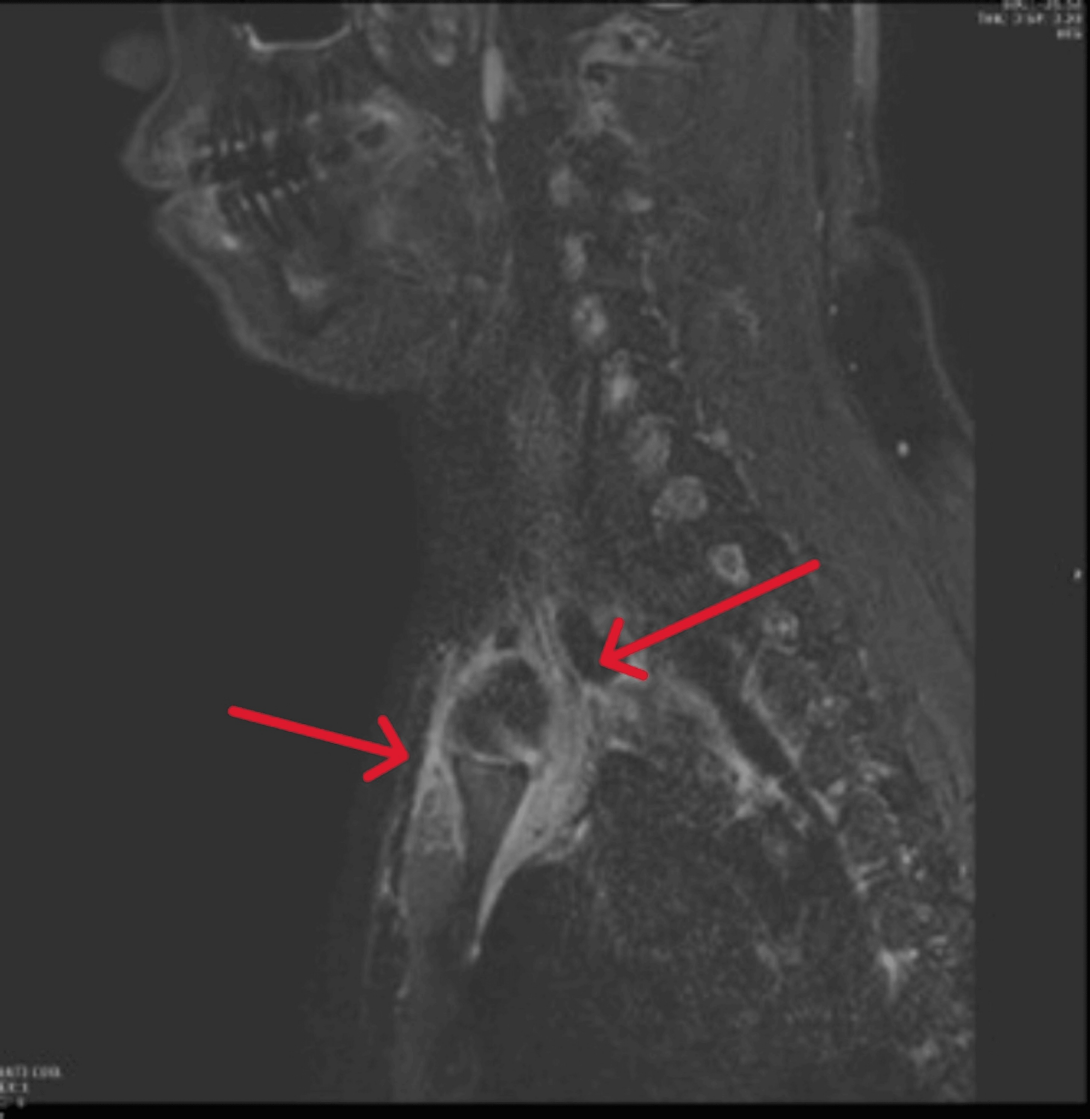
Sagittal short tau inversion recovery (STIR) MRI revealed deep soft tissue edema surrounding the sternoclavicular joints and the presternal and retrosternal soft tissues (red arrows).
